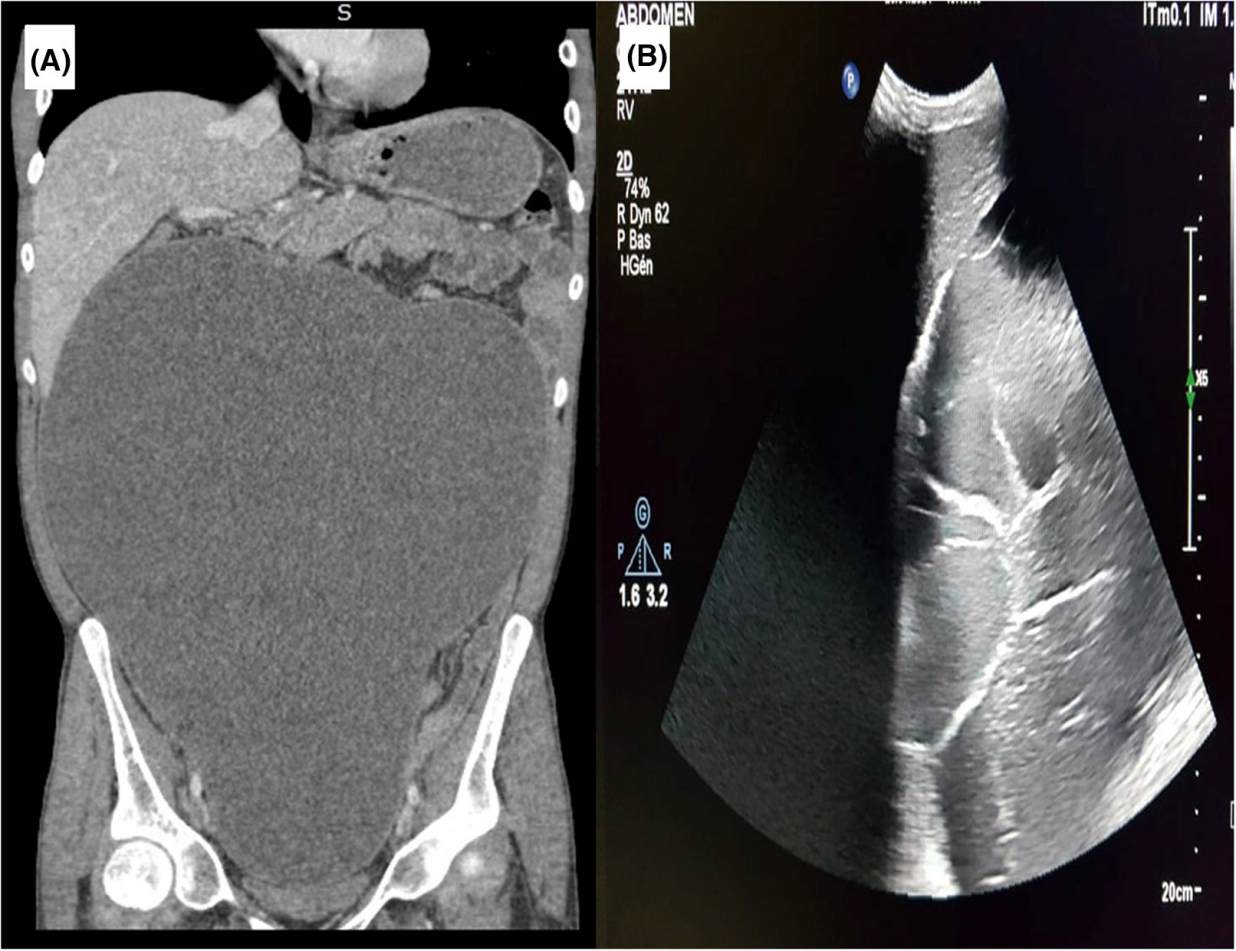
Radiological findings in abdominal ultrasound (A) and computed tomography (B): large abundance septated ascites
